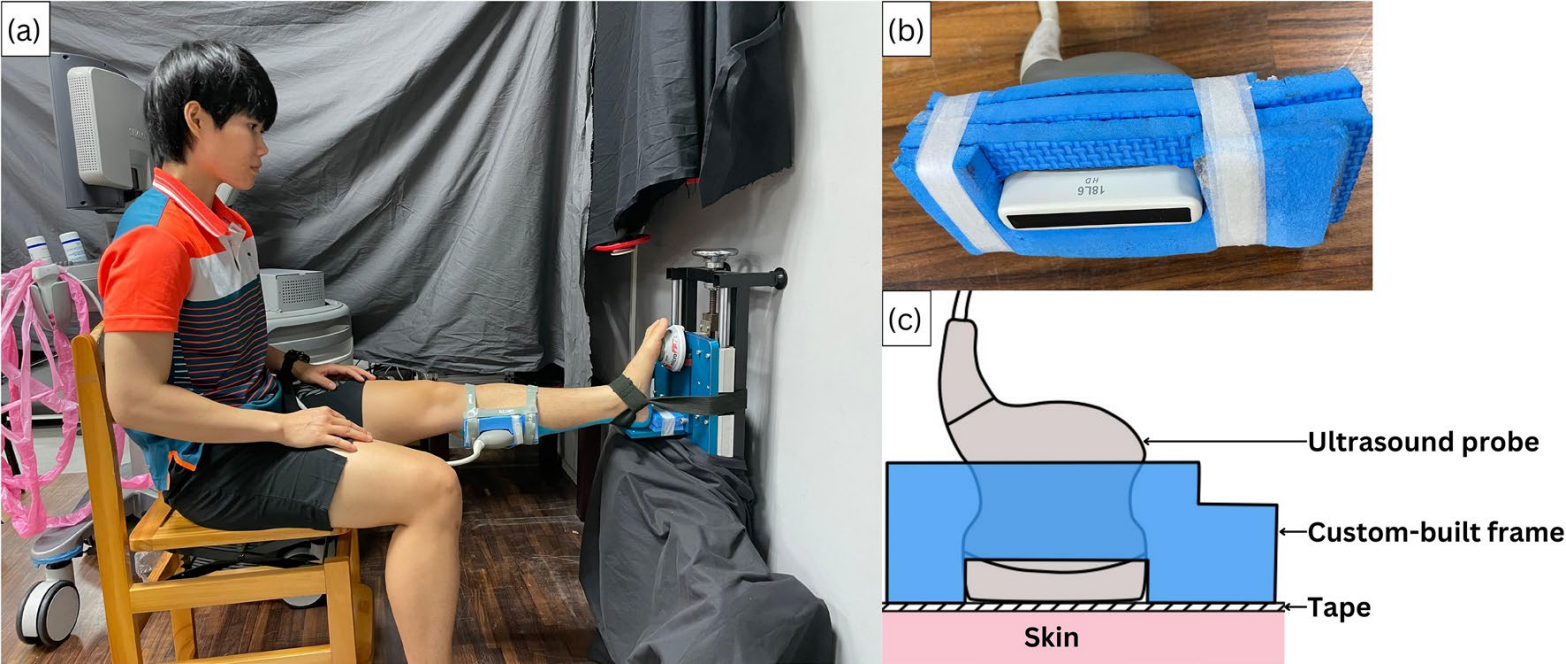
Maximal voluntary isometric plantar flexion task (a), the custom-built frame (b) and the cross-section view at the intersection of ultrasound probe and the tape (c).
